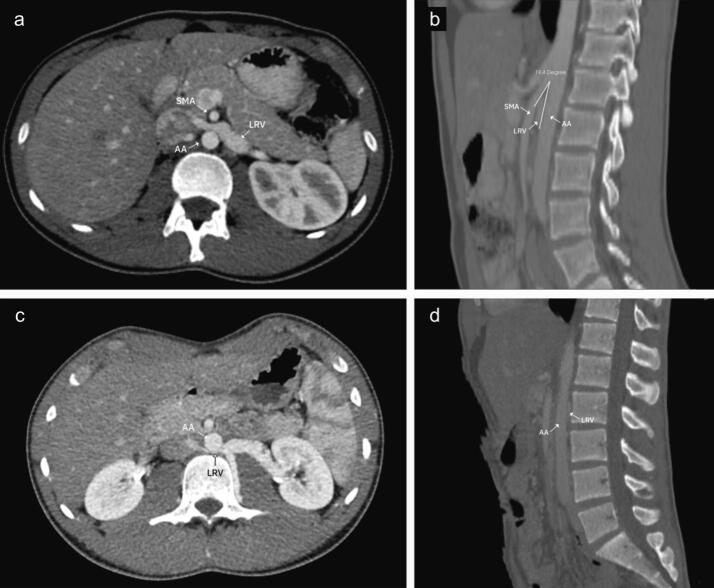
Preoperative Computed Tomography scan image of patients with anterior and posterior nutcracker syndrome (NCS). a) Anterior NCS - Axial view showing compression of the left renal vein (LRV) between the abdominal aorta (AA) and the superior mesenteric artery (SMA) (beak sign). b) Anterior NCS - Sagittal view showing the angle between SMA and AA is measured at approximately 19.4°. c) Posterior NCS - Axial view showing compression of LRV between AA and vertebrae body. d) Posterior NCS – Sagittal view.
